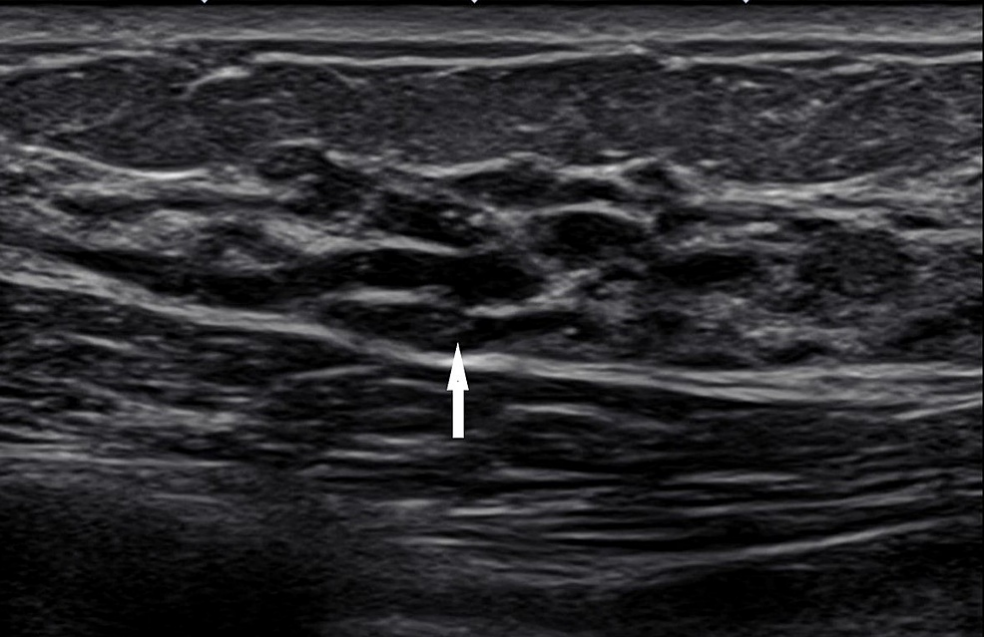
Grayscale transverse ultrasound image demonstrating dilated ducts/cystic appearance corresponding to the area of mammographic concern (white arrow).
